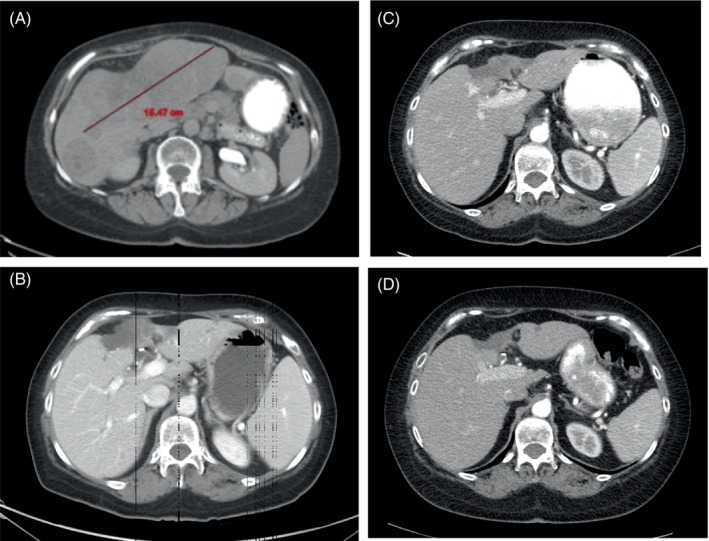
Computed tomography scans of hepatic metastases. (A) Initial presentation with largest diameter 15.5 centimeters. (B) Improvement of metastases at outside hospital after finishing treatment. (C) Continued improvement after 11 weeks from last treatment. (D) Continued improvement after 24 weeks from last treatment.
